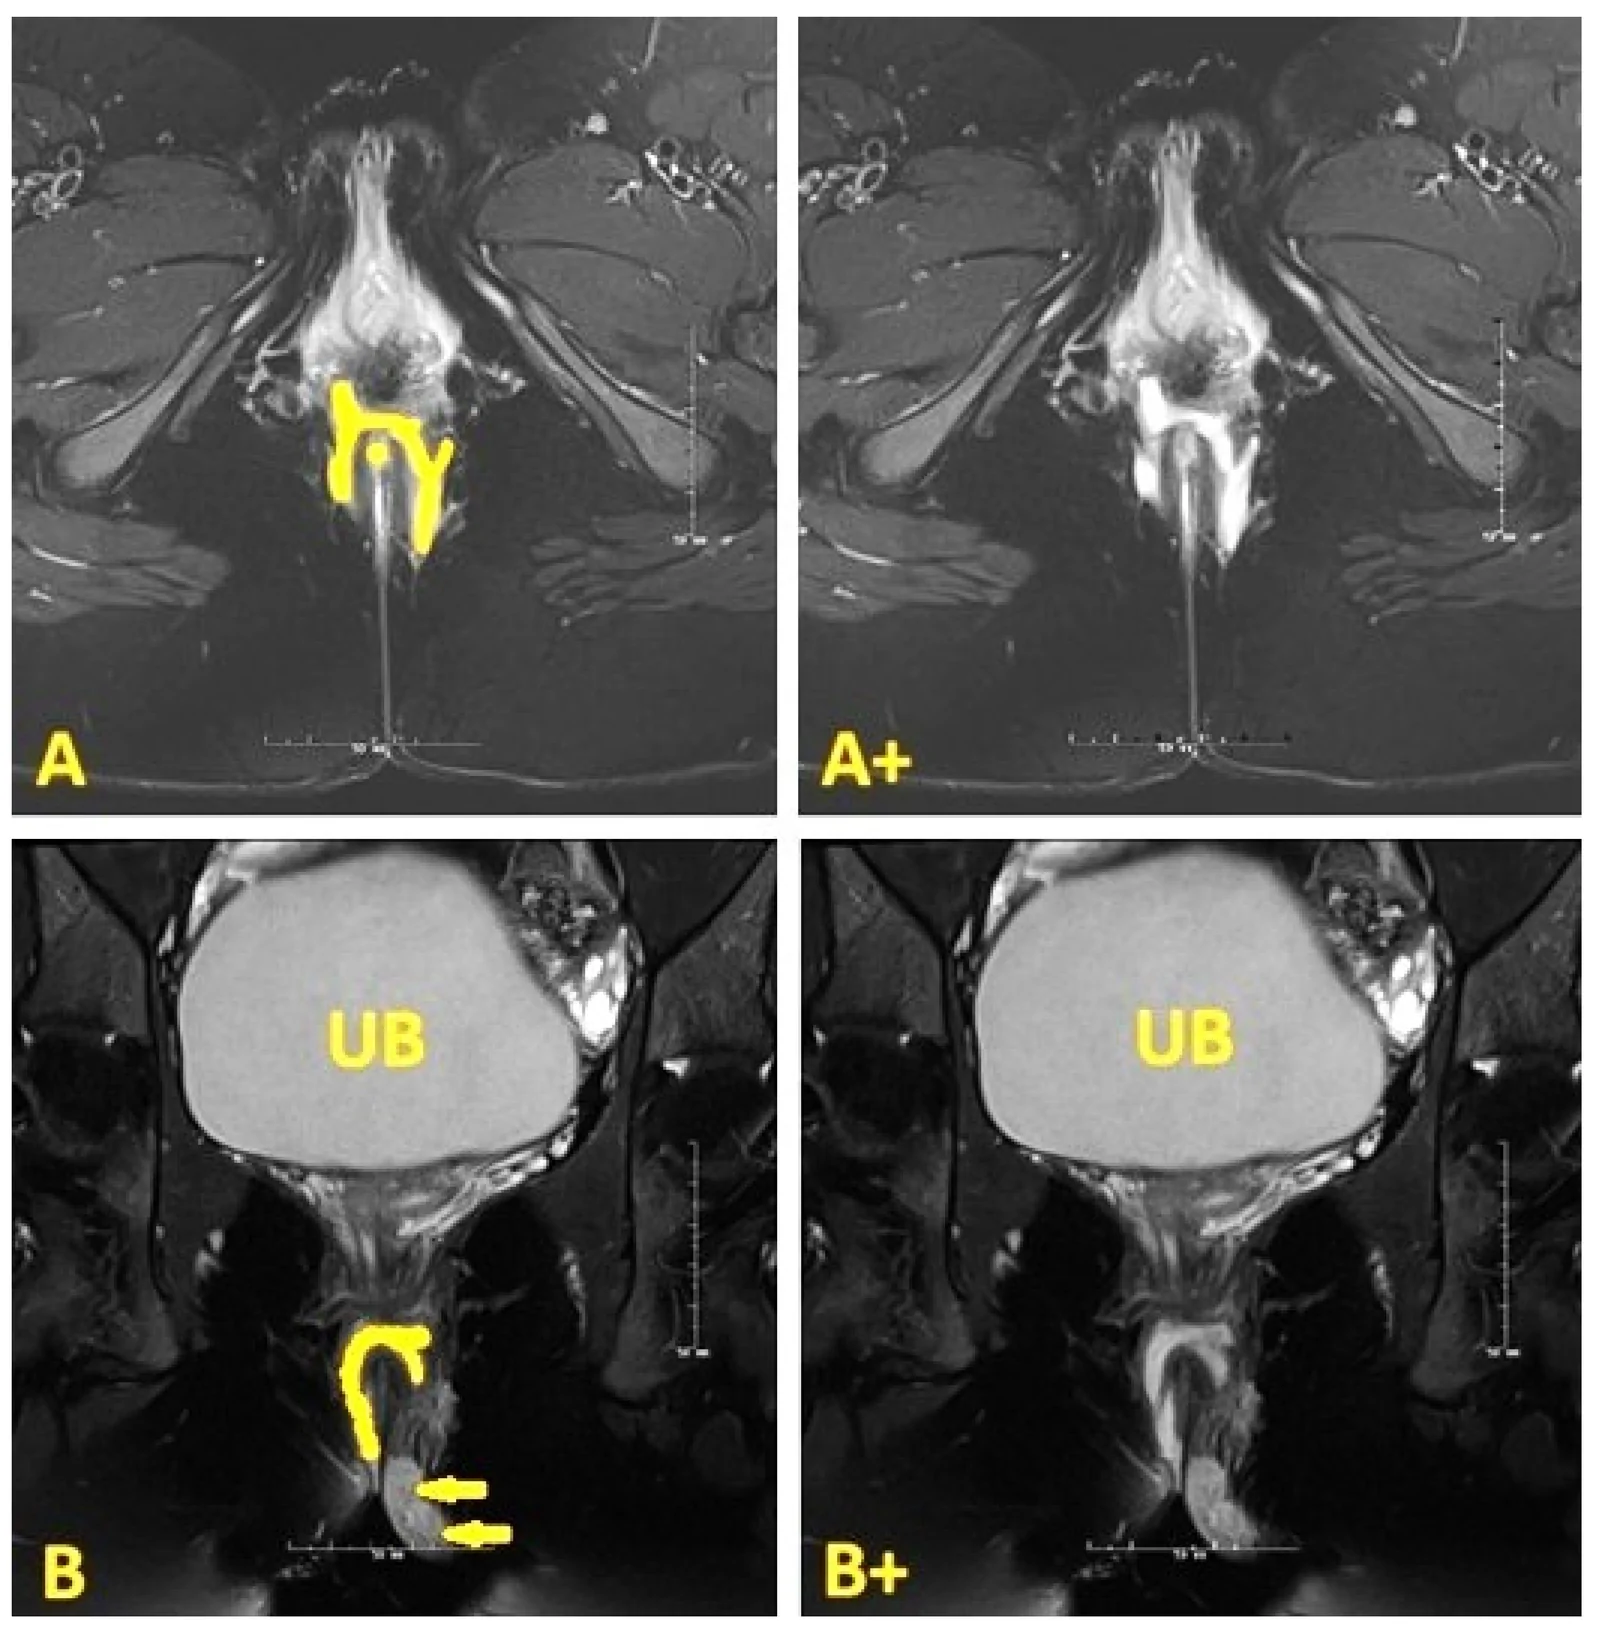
Selected magnetic resonance images of a patient with Crohn’s disease. (A) Axial fat-suppressed T2-weighted image showing a complex transsphincteric perianal fistula arising from the anterior midline anal sphincter region at the 12 o’clock position and extending into both sides of the perineum around the anal canal (dot), forming a horseshoe fistula. (A+) Corresponding unannotated image. (B) Coronal fat-suppressed T2-weighted image showing the complex fistula extending into both sides of the perineum, with greater involvement on the left side than on the right. Marked skin thickening and a hyperintense area are present in the left medial buttock, consistent with cellulitis (arrows). (B+) Corresponding unannotated image. UB: Urinary bladder.
